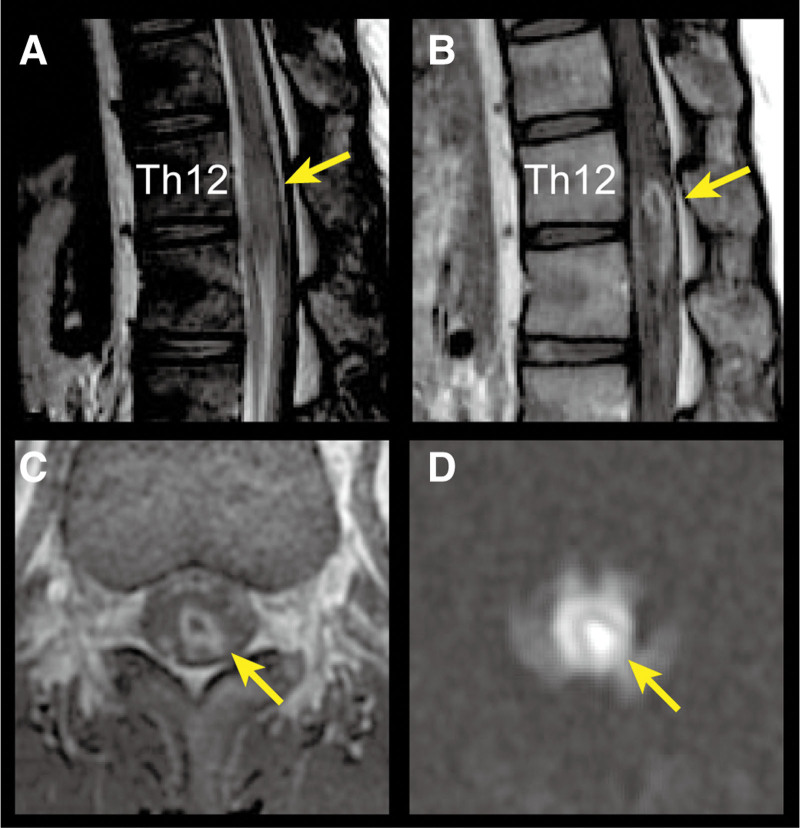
Spinal MRI. (A) Sagittal section of T2-weighed image of the thoracolumbar spine shows a high-intense area located at vertebral levels Th12–L2, associated with swelling of the spinal cord around the lesion, indicated by a yellow arrow. (B) Gadolinium-enhanced T1-weighted image shows enhancement around the lesion, indicated by a yellow arrow. (C) A ring-shaped gadolinium enhancement around the lesion in transverse section, indicated by a yellow arrow. (D) High-intensity lesion on diffusion-weighted image in transverse section, indicated by a yellow arrow. MRI = magnetic resonance imaging.
